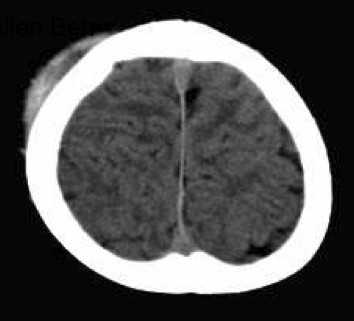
Head computed tomography, revealing subcutaneous hemorrhage without fracture or intracranial bleeding.
